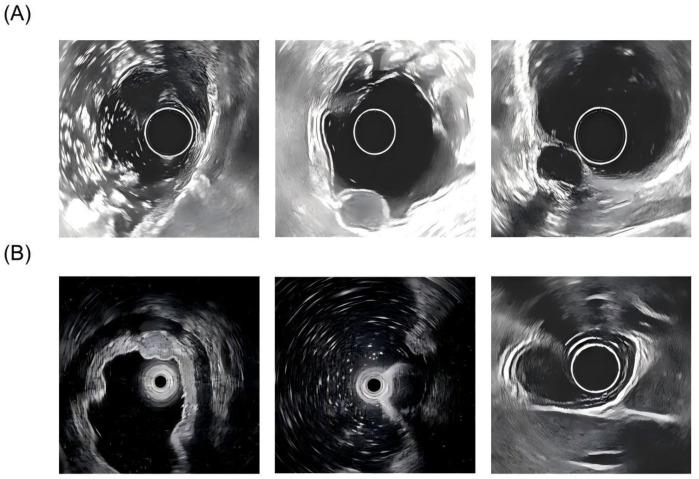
Representative endoscopic ultrasound images from the dataset. (A) Gastrointestinal stromal tumor. (B) Non-GIST lesions.
